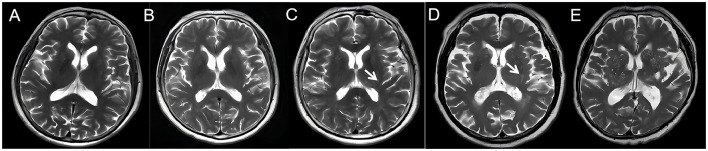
Axial T2-weighted MRI of the brain demonstrating BG-EPVS at different severity grades (0–4). (A) Grade 0 (B) grade 1 (C) grade 2 (D) grade 3 (E) grade 4. The white arrow points to EPVS.
